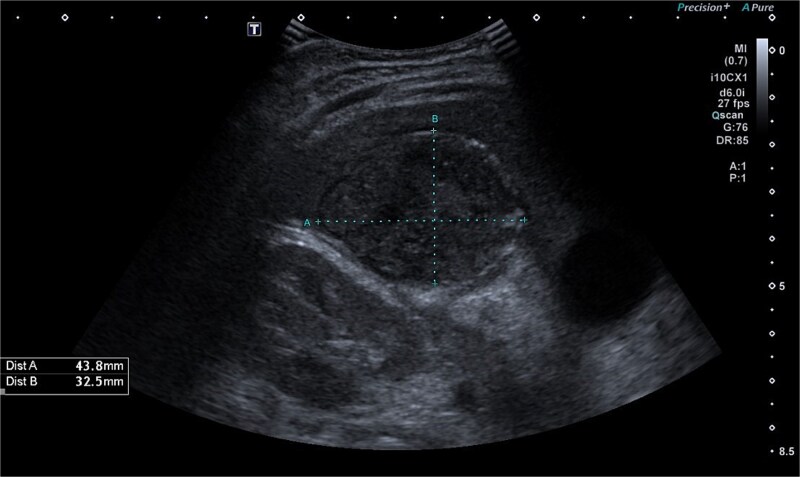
Abdominal ultrasonography imaging of pathological dense fluid collection adjacent to liver segment VI.
